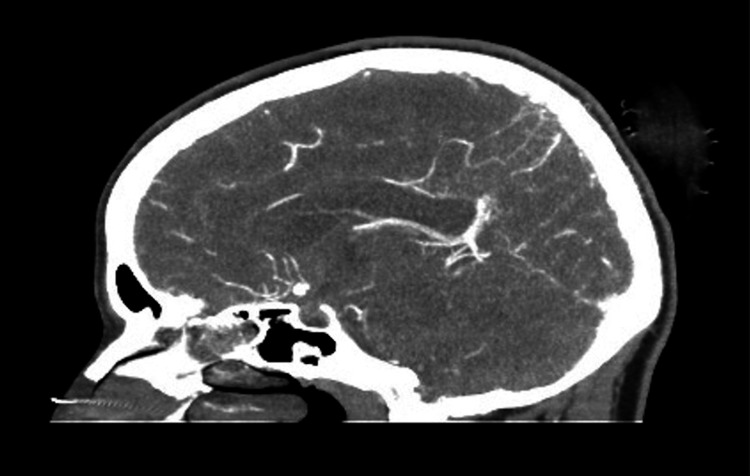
Sagittal computed tomography angiography with contrast
